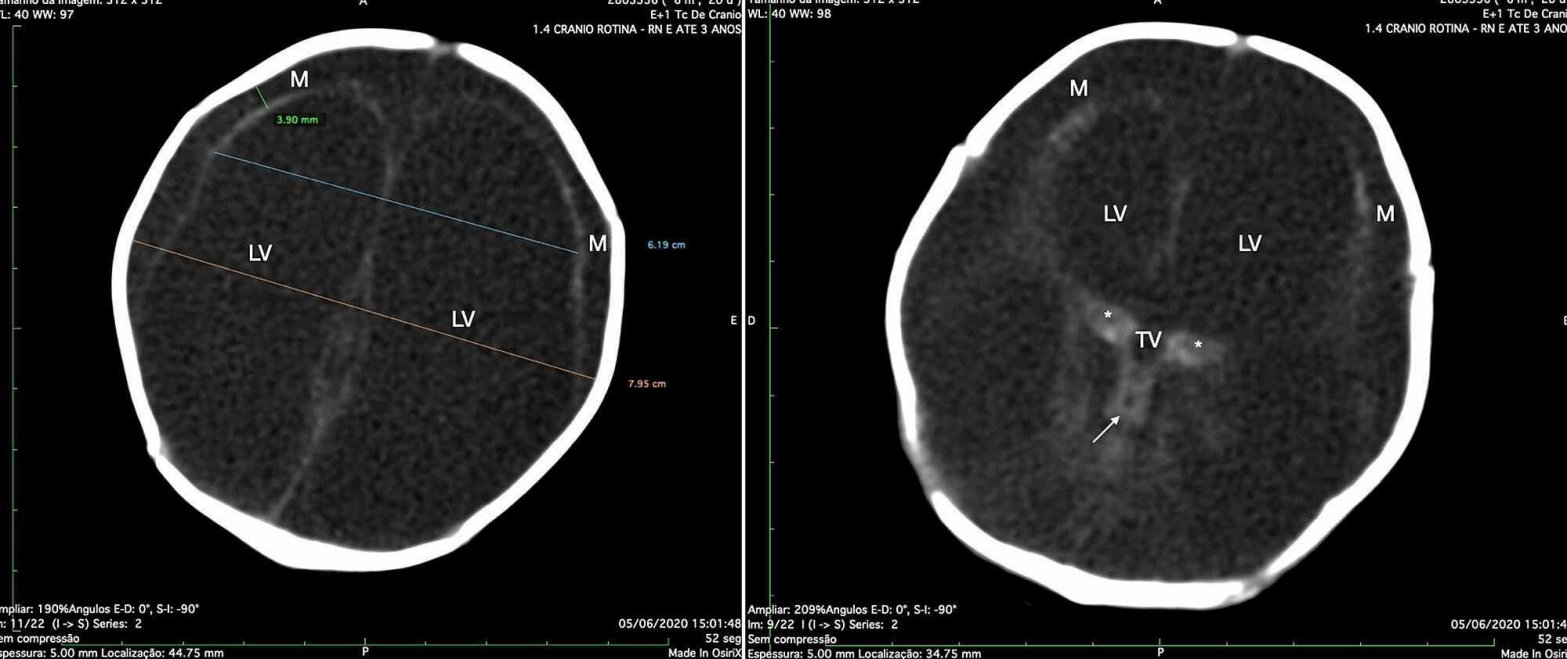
Brain image of a child with congenital brain injury before the head trauma CT scan of the brain without contrast of the patient at two months of age, showing a diffuse severe injury of the brain, presented as a hypodense parenchyma and thin cortical mantle (M) and enlarged compensatory LVs (Evans’ index = 0.77) (left). Thalamus (*), TV, and midbrain (white arrow) are identified (right). LV, lateral ventricle; TV, third ventricle.
